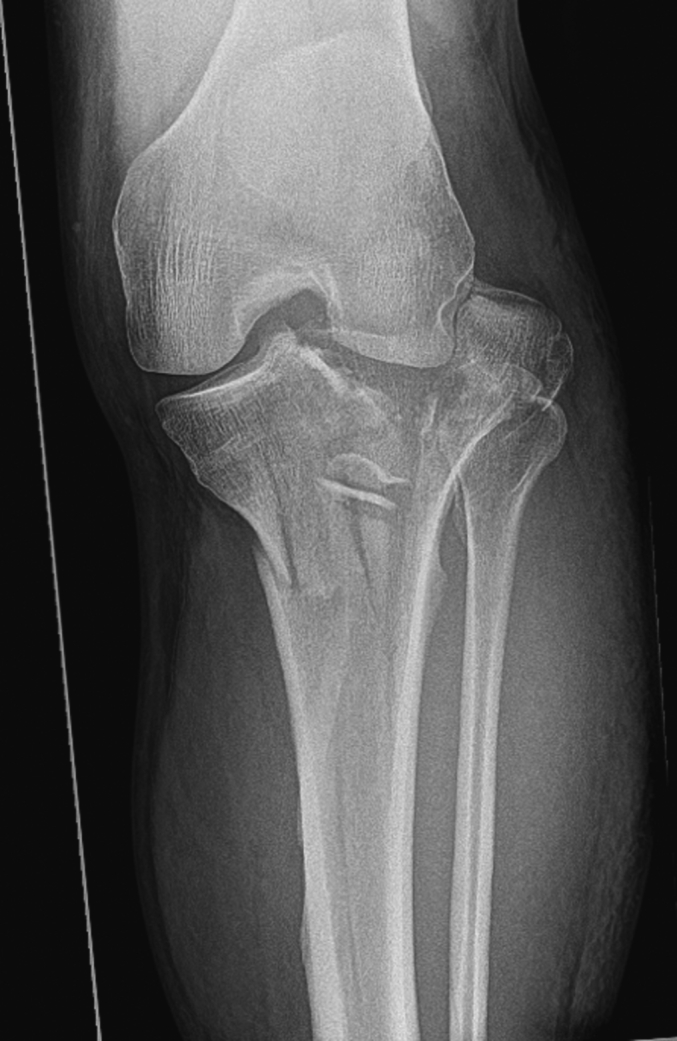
AP radiograph of a tibial plateau fracture-dislocation with partial loss of joint congruity.
